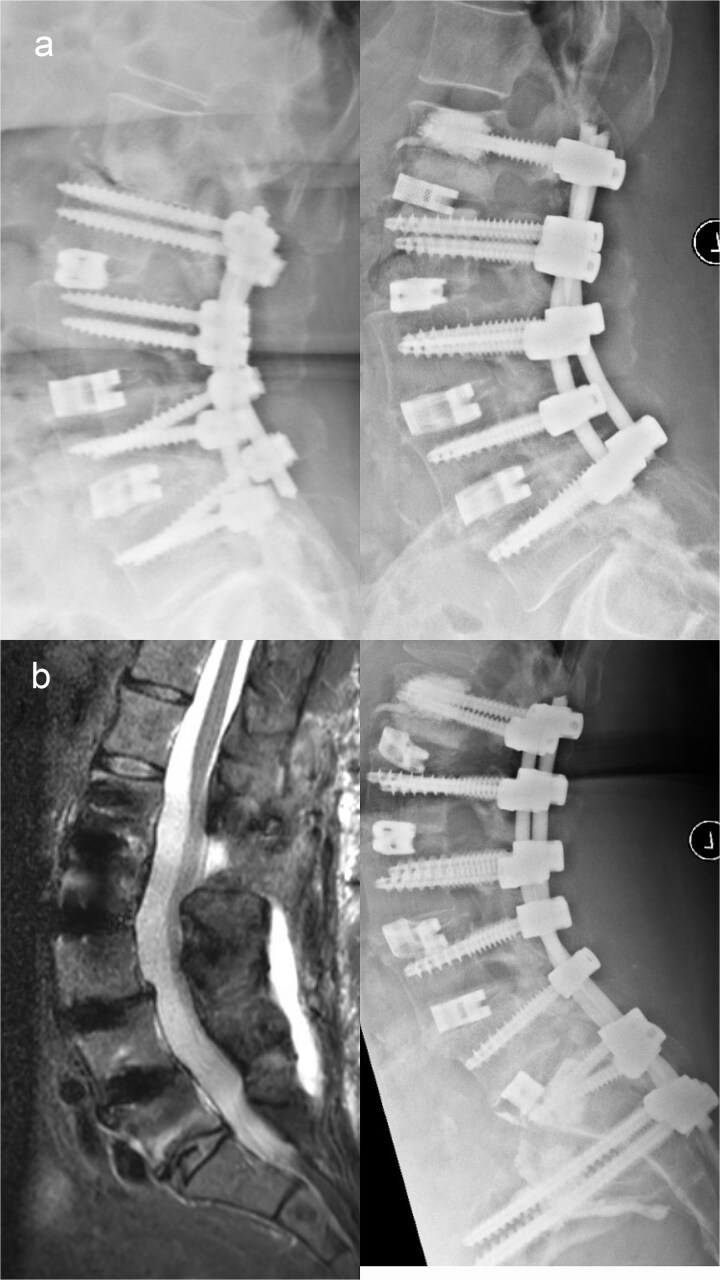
(a) Preoperative imaging demonstrating pronounced cranial adjacent segment degeneration at L1/2 with severe spinal canal stenosis and foraminal narrowing (left), and postoperative imaging following extension of the fusion to L1–L5 with left-sided TLIF at L1/2 (right). (b) Preoperative magnetic resonance imaging showing a fresh inferior endplate fracture of L5 (left), and postoperative imaging after extension of the spondylodesis to the ilium (right).
